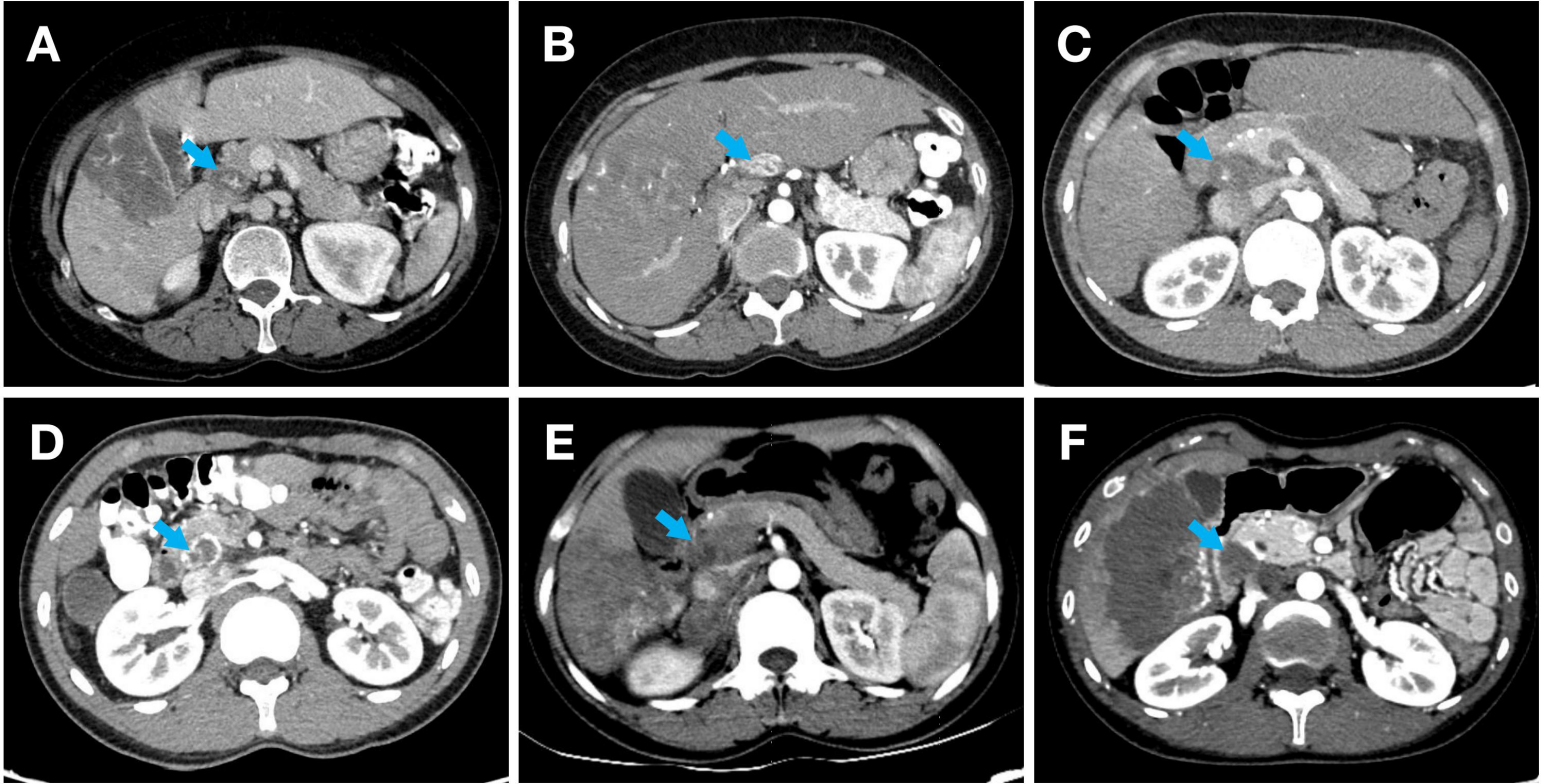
Contrast-enhanced CT scan features of metastatic LNs in HAE. (A) An enlarged LN with a diameter of 23 mm beside the portal vein, nodular calcification and low-density areas within the lesion, and heterogeneous enhancement on an enhanced scanning. (B) An enlarged LN with a diameter of 21 mm beside the common hepatic artery, sand-like calcifications within the lesion, and heterogeneous enhancement on enhanced scanning. (C) Enlarged and fused LNs with a diameter of approximately 39 mm behind the pancreatic head and in front of the inferior vena cava, nodular calcification within the lesion, and homogeneous enhancement on enhanced scanning. (D) Enlarged and fused LNs with a diameter of approximately 23 mm behind the pancreatic head and in front of the inferior vena cava, sand-like and eggshell-like calcifications around the lesion, and heterogeneous enhancement on enhanced scan. (E) Enlarged and fused LNs with a diameter of approximately 44 mm behind the pancreatic head and in front of the inferior vena cava, patchy slightly low-density shadows within the lesion, heterogeneous enhancement on enhanced scan, and patchy non-enhanced liquefactive necrotic areas within the lesion. (F) Enlarged and fused LNs with a diameter of approximately 23 mm behind the common bile duct, eggshell-like calcifications around the lesion, moderate enhancement at the edge of the lesion on enhanced scanning and no obvious enhancement within the lesion.
